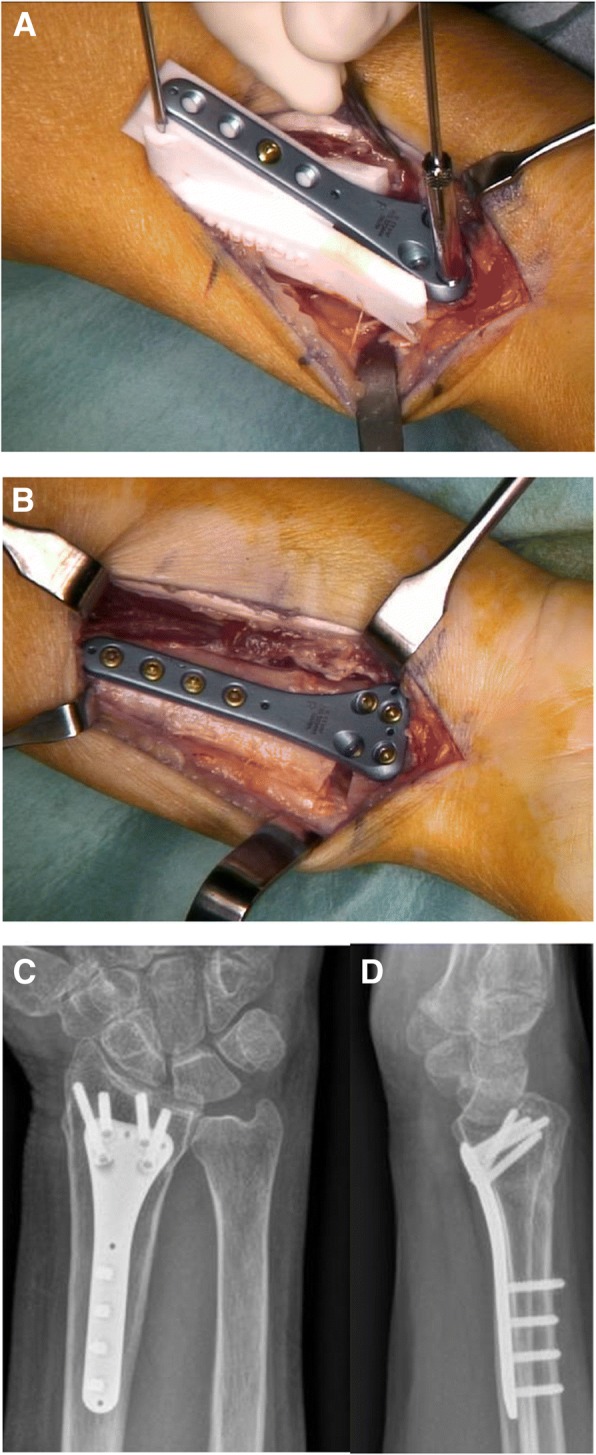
a Ramp-guide (white) with temporal fixation by K-wires on the most proximal drilling-hole. b Plate reduction with fixated distal fragment after guide removal. c and d Posteroanterior and lateral radiographs after corrective osteotomy
